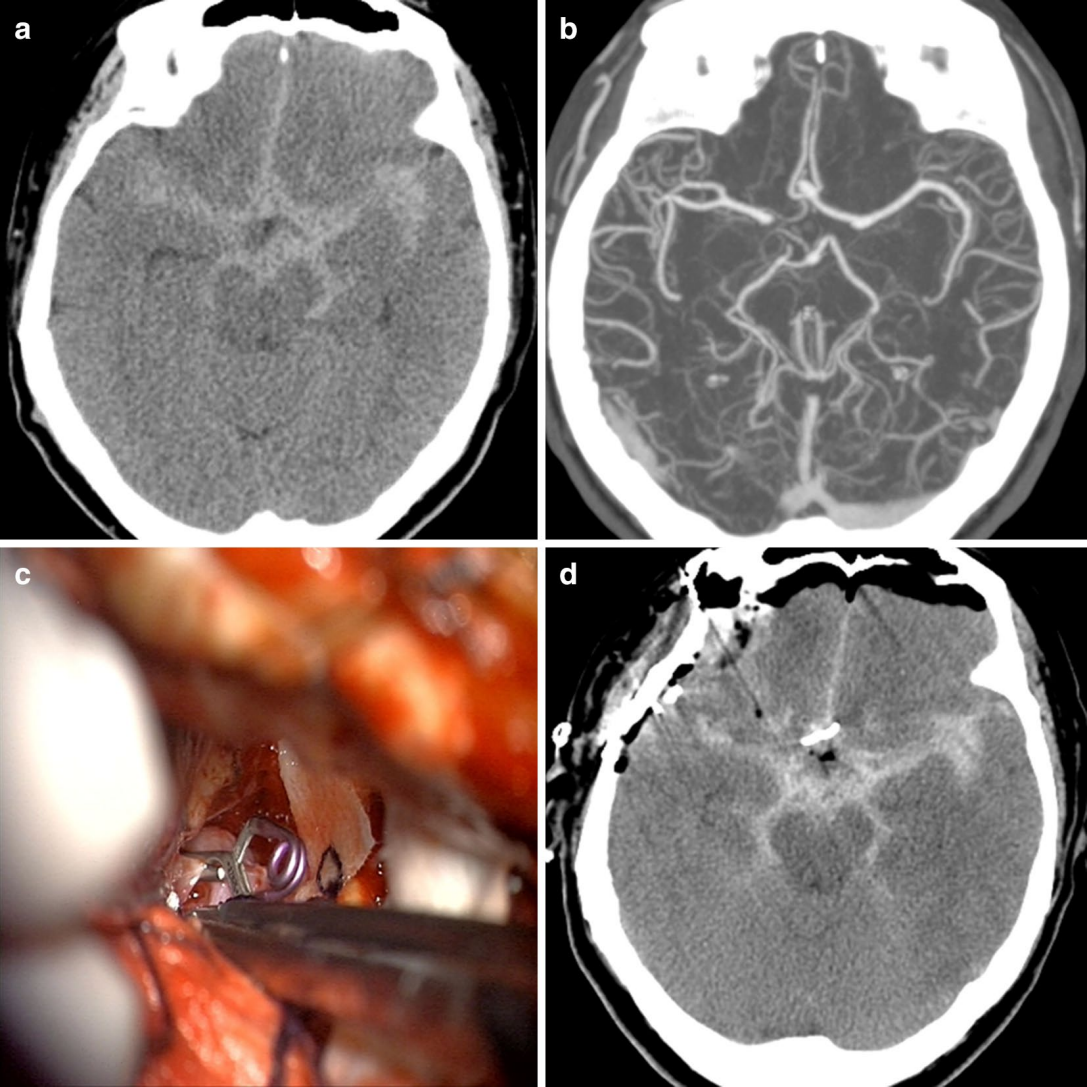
Axial non-contrast CT scan showed diffuse SAH in the basal cisterns, anterior interhemispheric fissure and sylvian fissures (a). CT angiography demonstrated ruptured aneurysm of the anterior communicating artery (b). Intraoperative photograph and post-operative CT scan showed the clipped aneurysm without any complications (c, d)
